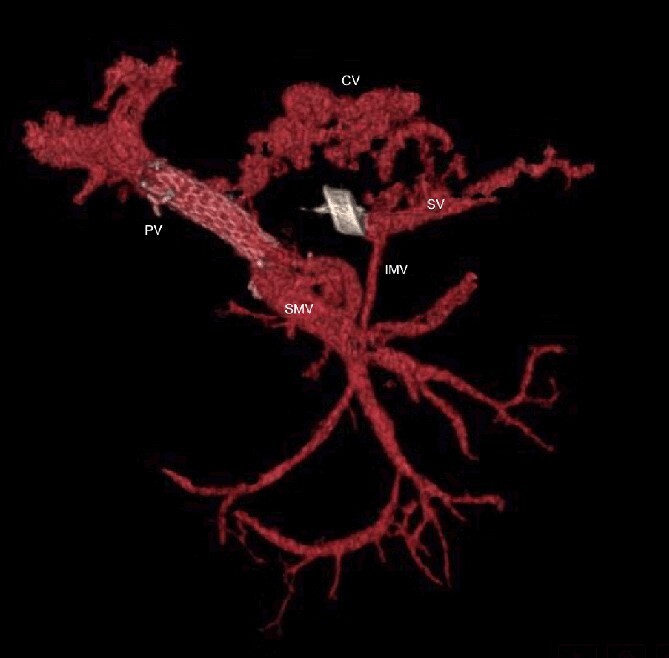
CT scan at 3 years, with development of venous collaterals that drain distal splenic vein and inferior mesenteric vein through the lesser omentum. PV portal vein, SMV superior mesenteric vein, SV splenic vein, IMV inferior mesenteric vein, CV collateral veins.
